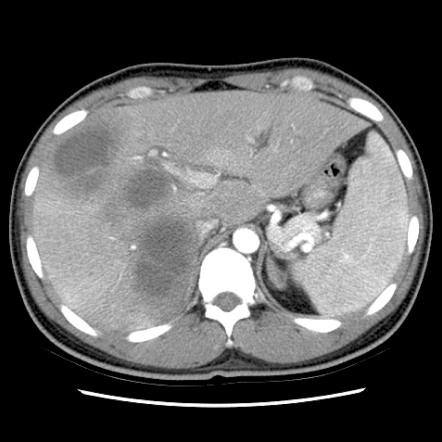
Abdominal computed tomography showing multiple liver abscesses of a 28-year-old male homosexual who presented with right upper quadrant pain, vomiting, and watery diarrhea for 4 days. Diagnosis of amebic liver abscess was confirmed by positive PCR for *Entamoeba histolytica* of the liver abscess aspirate. The titer of indirect hemagglutination antibody for *E. histolytica* was 8192.
